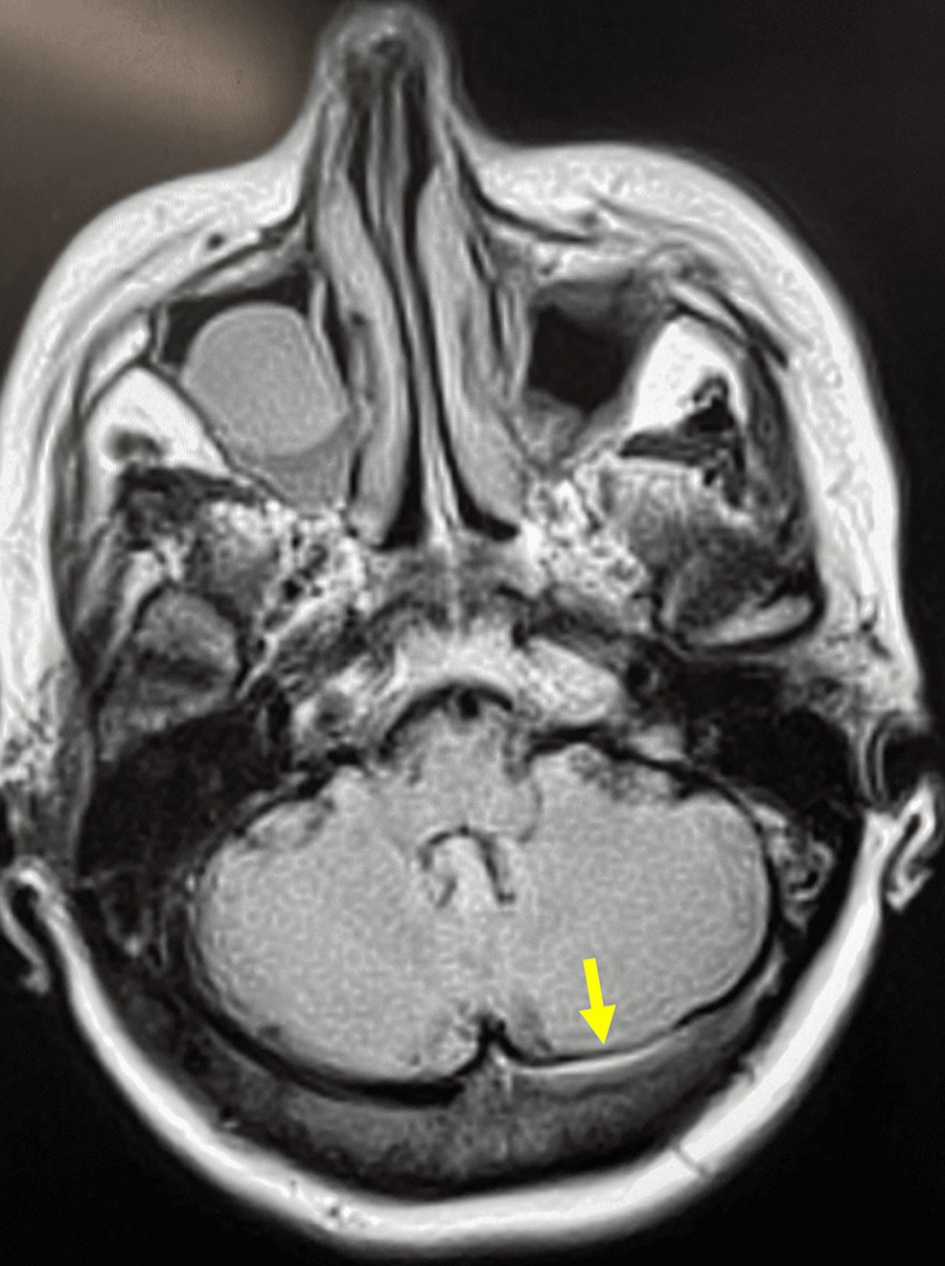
MR imaging with features indicating CVST. MR: magnetic resonance, CVST: cerebral venous sinus thrombosis
